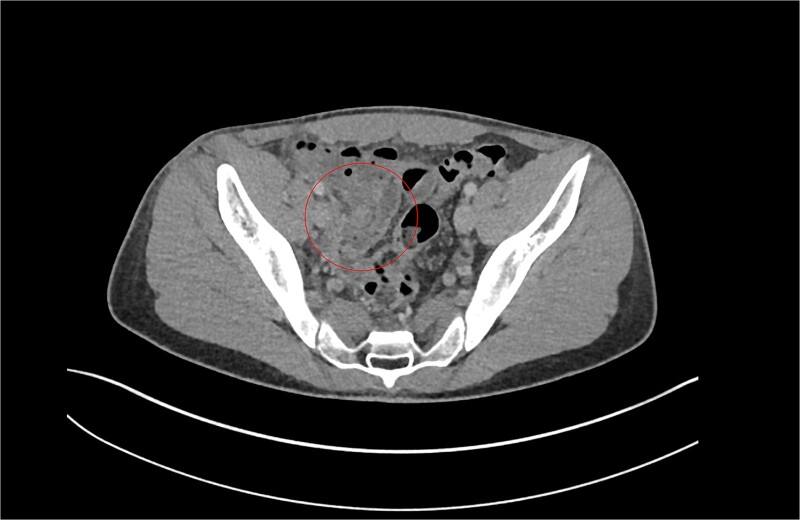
Patient B. Appendiceal stump of Patient B identified within the red circle on CT scan before the second operation.
